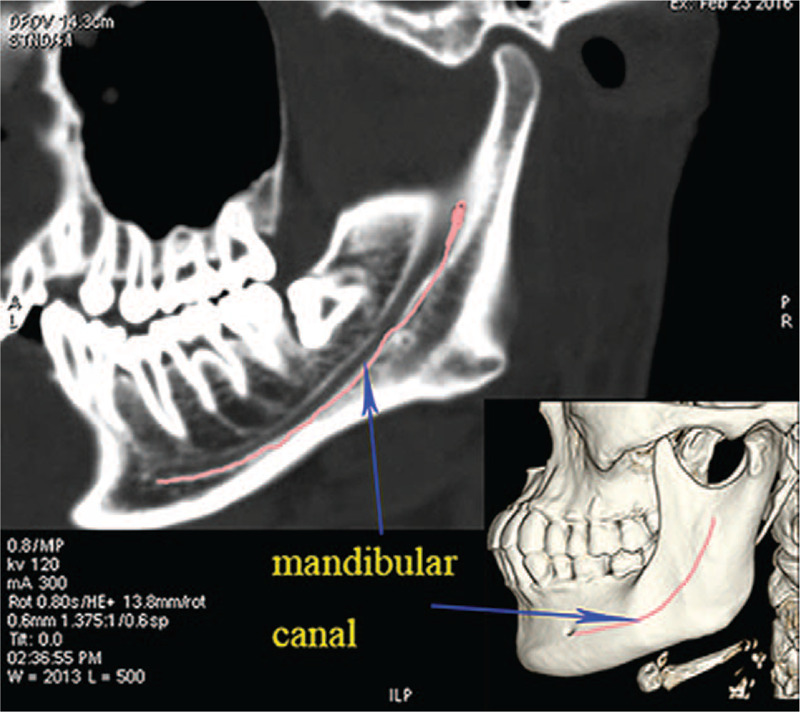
Real-time mark of the mandibular canal in the reconstructed CT image and the 3D skull model.
